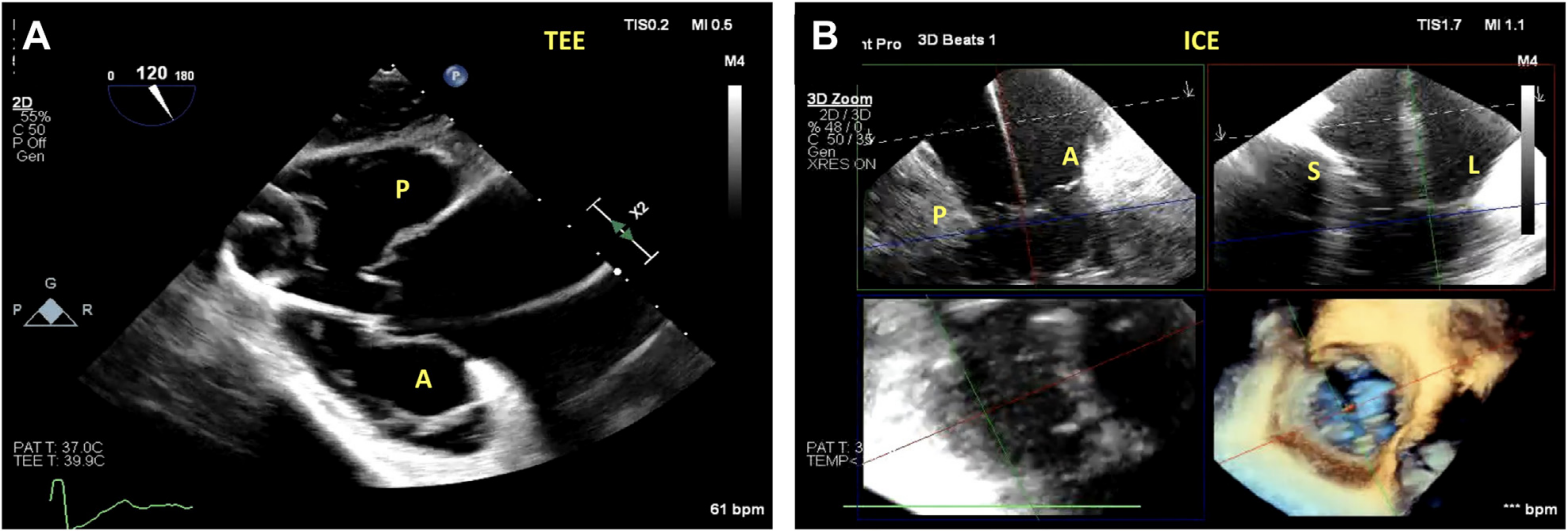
Guiding Wire and Delivery System (A) Gastric image on transesophageal echocardiography (TEE) demonstrating wire placement with the curl of the wire facing posteriorly. (B) 3D MPR on ICE is then visualized to guide the delivery system into the RV considering the anterior, posterior, septal, and lateral orientation of the device. Abbreviations as in Figures 1 and 2.
